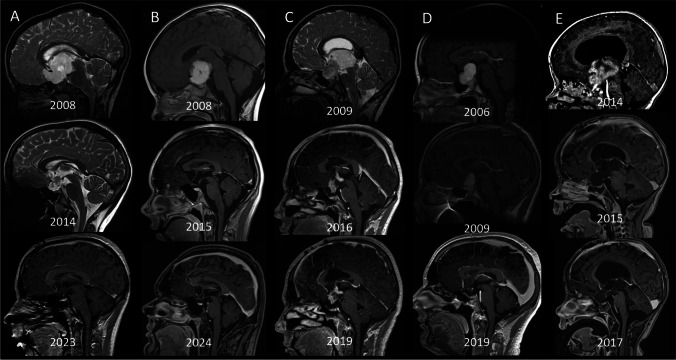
Case examples with long-term follow-up representing the multimodal treatment approach in CHG including relevant surgical strategies. A A 4-year-old boy presented with visual impairment had a biopsy. Neuropathology revealed pilocytic astrocytoma subtype pilomyxoid WHO I°. Standard LGG chemotherapy regimen (carboplatin/VCR) resulted in relevant decrease of the tumor mass although other systemic treatment lines needed to follow. Visual acuity at follow-up: right 0; left 1.0. B A 4-year-old boy presenting with nystagmus showed relevant tumor mass with exophytic tumor components. Staged debulking surgeries were scheduled and one chemotherapy was administered finally reaching stable disease. C A 9-year-old boy presented with nausea and vomiting. MRI revealed a hydrocephalus and a CHG. An interhemispheric trans-callosal resection of T2 hyperintense, 3rd ventricular part was performed twice during disease, eventually reaching stable disease. D A 12-year-old boy with visual impairment was initially treated through a subfrontal lateral approach. After developing further progression at the age of 15 years, another debulking was achieved followed by radiation. Visual acuity at follow-up: right 0.4; left 1.0; visual field assessment revealed incomplete hemianopia; E A 4-year-old boy suffering from headache and irritability had trans-callosal debulking surgery. Despite the freed 3rd ventricle, ongoing hydrocephalus required VP shunt implantation and MRI showed secondary tumor dissemination. Despite multiple lines of chemotherapy-refractory tumor progression was observed, and the patient died at the age of 7 years
